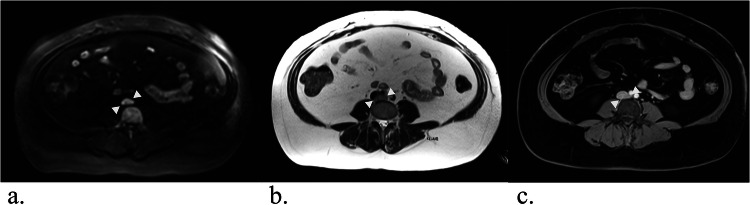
Whole-body MRI of a 34-year-old female study participant with LFS shows a progressive mass behind the inferior vena cava (white arrows). It is very clearly visible in diffusion-weighted imaging (a, b-value 900). In the HASTE sequence (b), the lesion is somewhat less conspicuous but visible, especially with prior knowledge. The lesion was classified as “easily missed” in the contrast-enhanced T1-weighted Dixon sequence (c, here: water-only image), as it was barely distinguishable from the adjacent inferior vena cava. Biopsy revealed a leiomyosarcoma. Images were cropped for better visibility of the lesion
